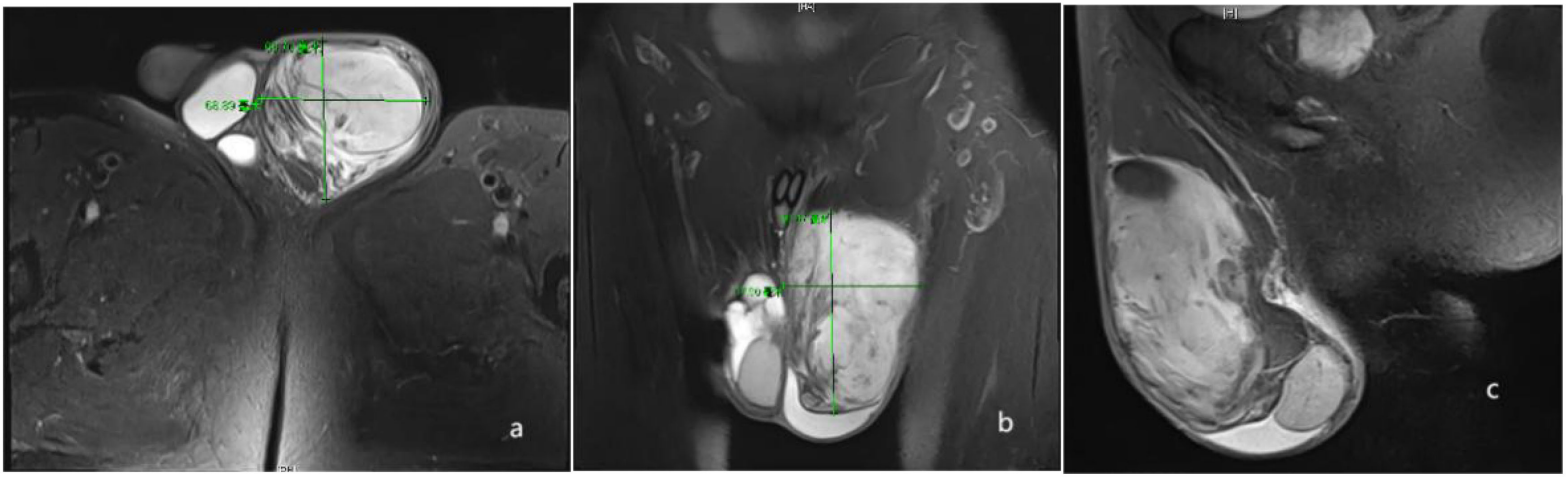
Transversal (a) coronal (b) and sagittal (c) MRI scan of the mass of the left spermatic cord. MRI indicated that the volume of the left scrotum was enlarged, revealing an isotropic T1 and mixed long T2 signal mass within. The borders of the mass were well-defined, measuring approximately 67 x 56 x98 mm. Additionally, multiple strips of short T2 signals were observed within the mass, accompanied by mild localized diffusion restriction on DWI and ADC maps. The left testis appeared compressed, and some pelvic adipose tissue had herniated into the scrotum through the left inguinal canal.
